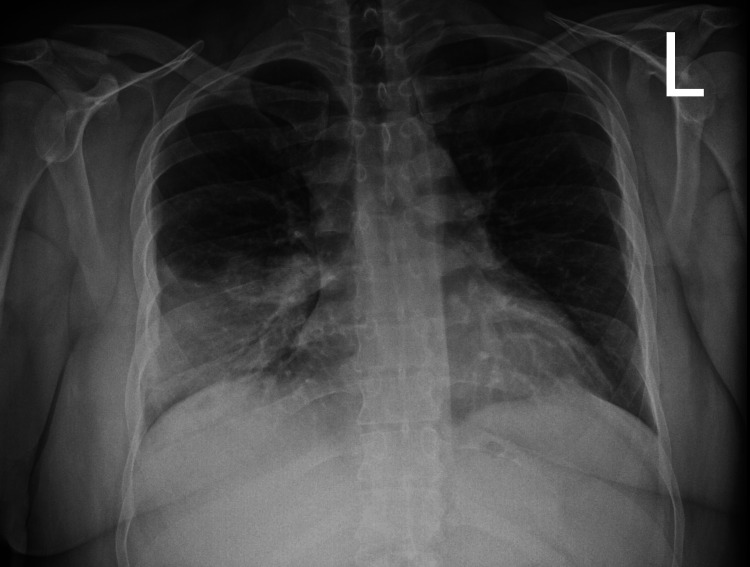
AP chest X-ray showing incidental lower lobe consolidation at initial presentation despite only complaining of abdominal pain and chronic diarrhea.
